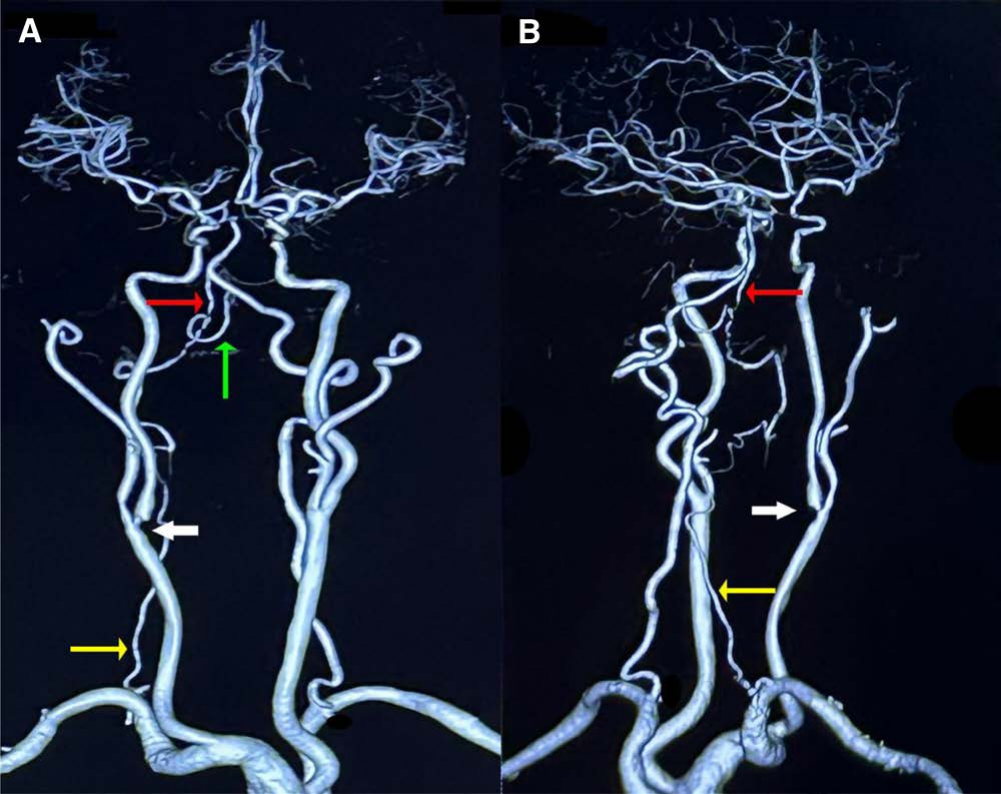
(A and B) The CTA revealed severe stenosis at the origin of the right internal carotid artery (white arrow) and occlusion of the right vertebral artery. The right deep cervical artery (yellow arrow) was connected to the V2 segment of the right vertebral artery (red arrow), and a prominent right PICA was observed (green arrow). The left vertebral artery and the left internal carotid artery appeared normal. CTA = computed tomography angiography, PICA = posterior inferior cerebellar artery.
